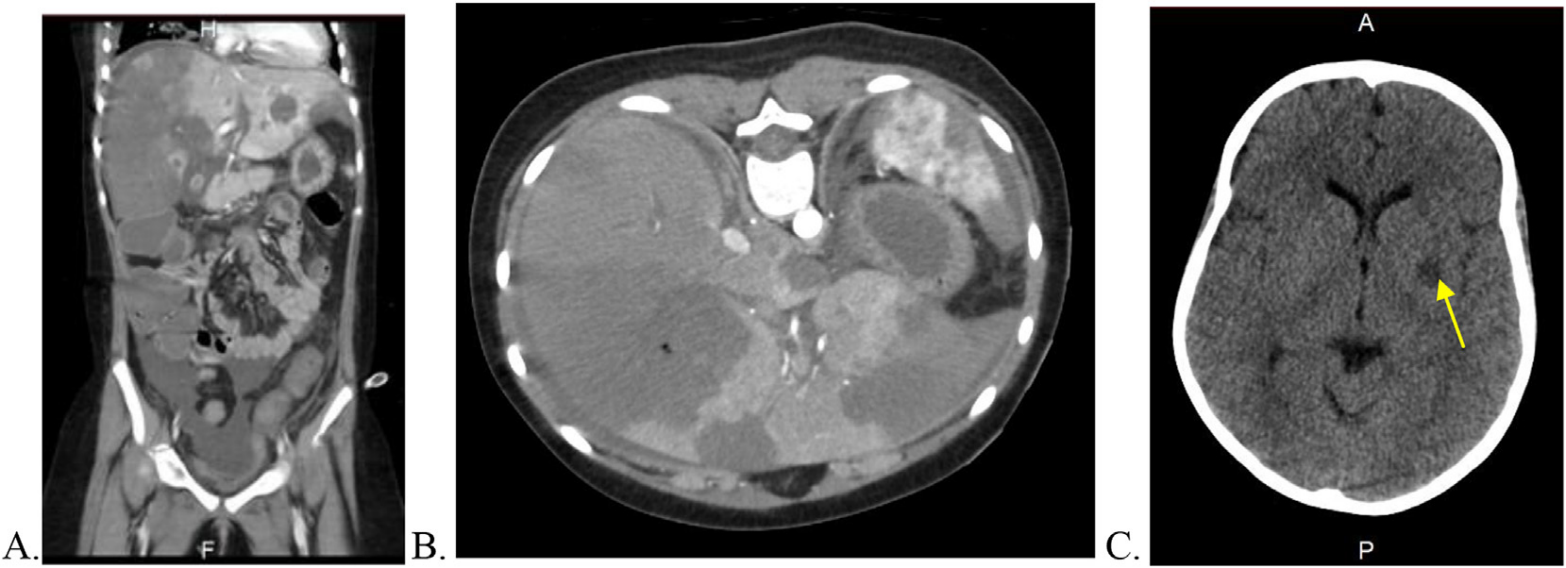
Computed tomography (CT) scan in case 3. A: Coronal section of CT abdomen showing multiorgan infarction involving multiple segments of the liver, the spleen, left kidney, and gall bladder. B: Horizontal section of CT abdomen again showing liver, spleen and kidney infarction. C: CT head with brain infarction in the left corona radiata and lentiform nucleus measuring 1.0 × 1.3cm (Arrow).
